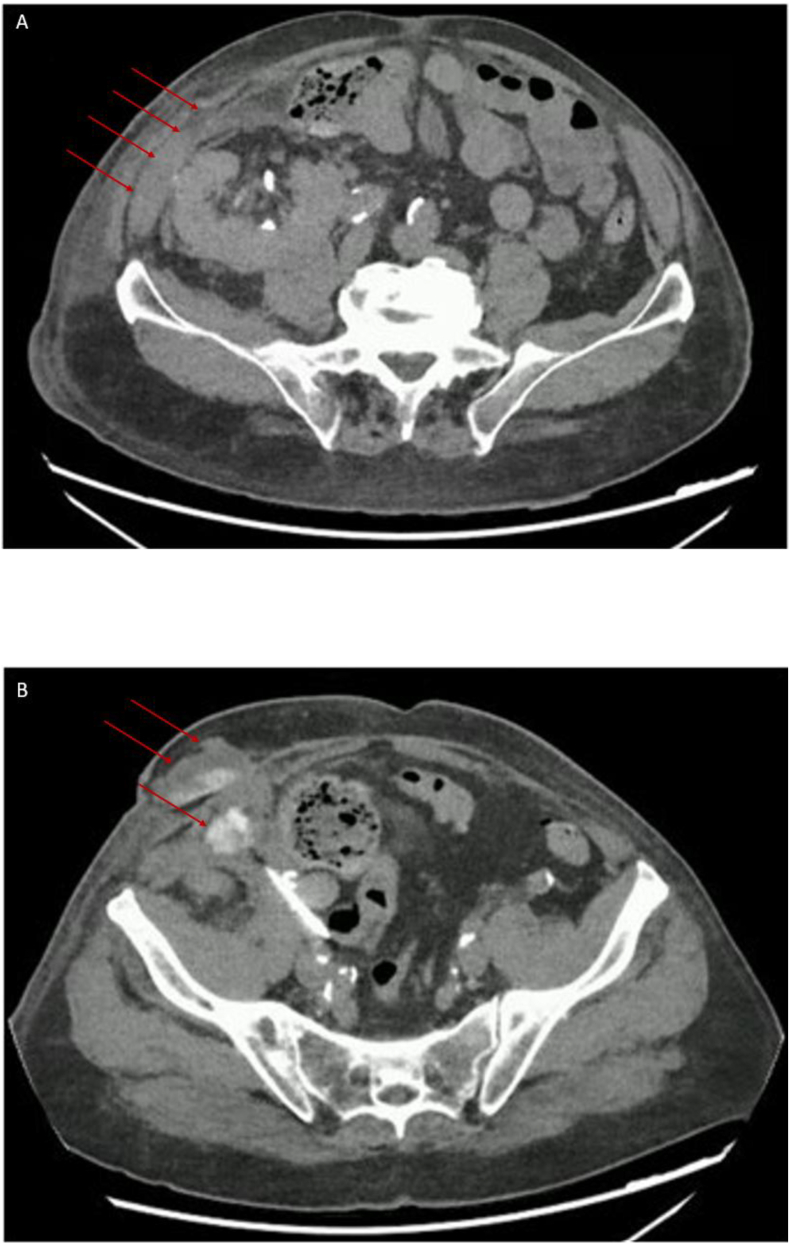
Baseline CT-scan revealing a urinary fistula despite nephrostomy and monoJ-stent drainage (A) Arrows demonstrate a urinoma on the anterolateral side of the renal graft; (B) Arrows demonstrate a urinoma extending into the anterior abdominal wall and forming a fistula through the surgical wound.
